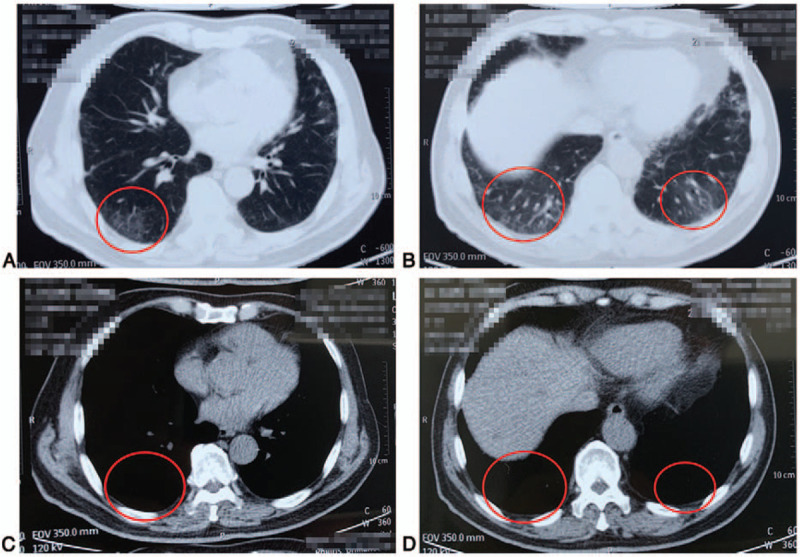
(A–D) CT examination after prednisone therapy indicated complete resolution (red circles) of the bilateral lung lesions.
